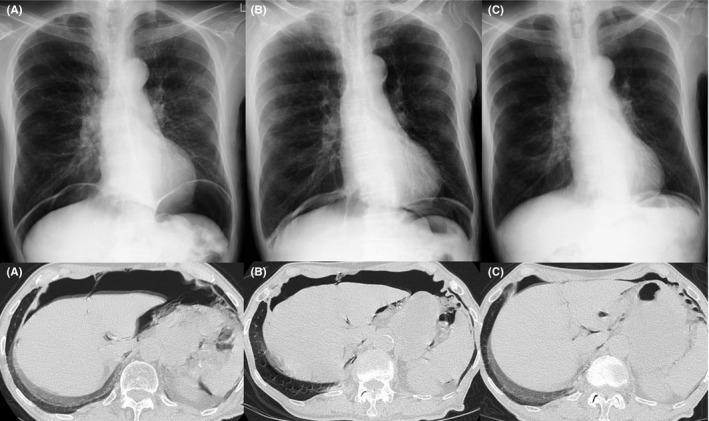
Radiological examination of Case 2. Chest X‐ray and computed tomography showed pneumoperitoneum spontaneously decreased. A, January 2014. B, March 2014. C, August 2014
